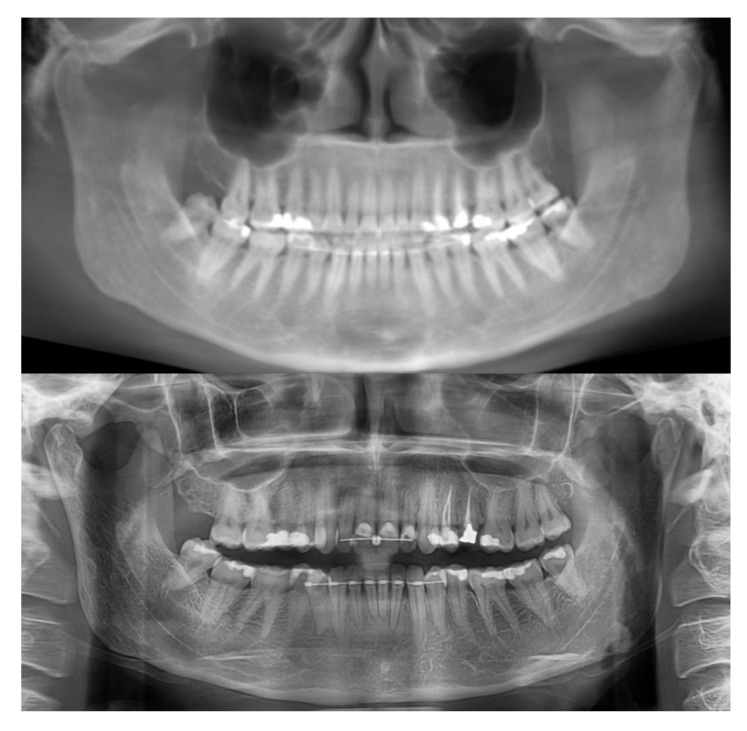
Pan-like reconstruction from CBCT volume after axial re-orientation and creating agreement with conventional pan.
